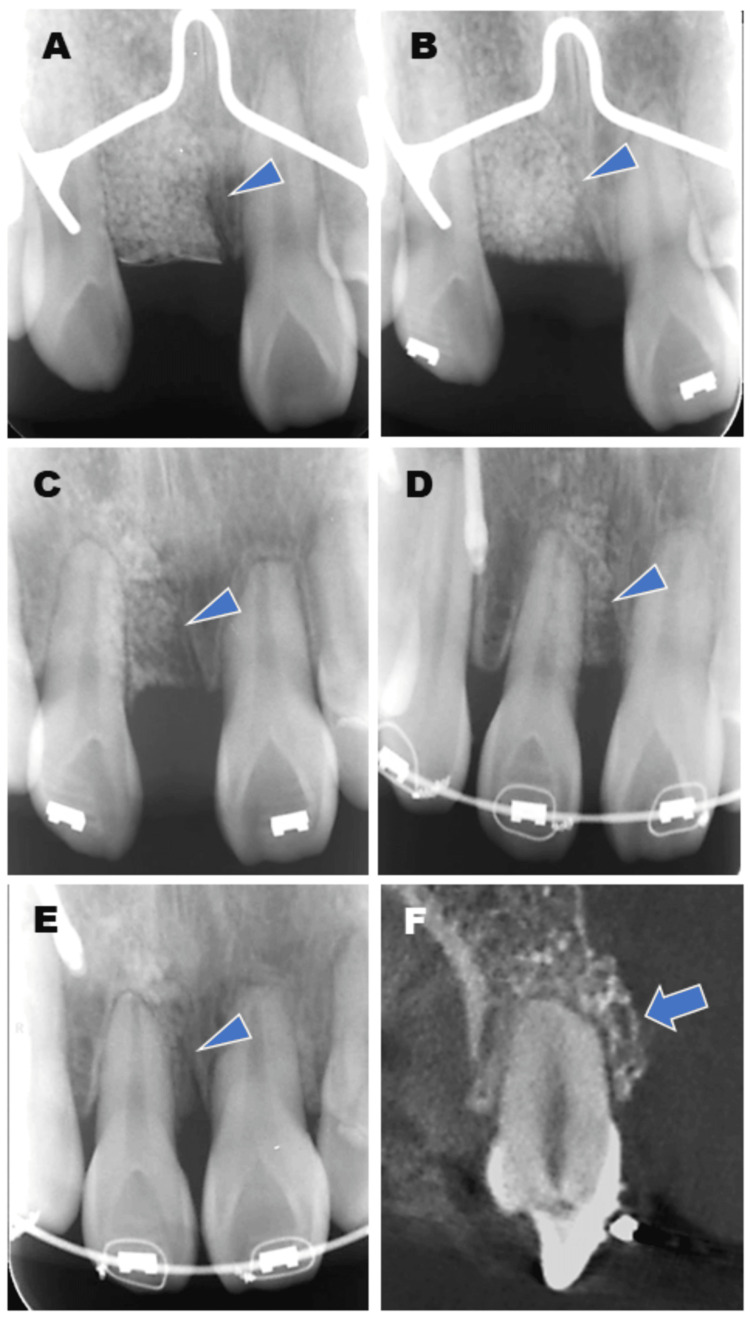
Time course observation of tooth movement by radiographs. (A) Dental X-ray image immediately after bone grafting with CO₃Ap granules. (B) Six months after the bone grafting, the border between the granules and the existing bone is becoming unclear. Orthodontic tooth movement (OTM) was started. (C) Two years after OTM, although small amounts of granules remain, alveolar bone with a well-defined structure is observed around the moving tooth. (D) Two years and eight months after OTM, tooth movement into the augmented area was completed. No abnormal findings were observed around the root of the tooth. (E, F) Dental X-ray and CBCT images one year after the completion of OTM. Mature alveolar bone was observed around the root. Arrowheads indicate residual granules, showing their gradual resorption and replacement with newly formed bone, and also highlight areas of bone remodeling in response to orthodontic forces. Arrows show sufficient labial bone volume, which is important for ensuring the stability of the tooth after orthodontic movement. CO₃Ap, carbonate apatite; CBCT, cone-beam computed tomography
